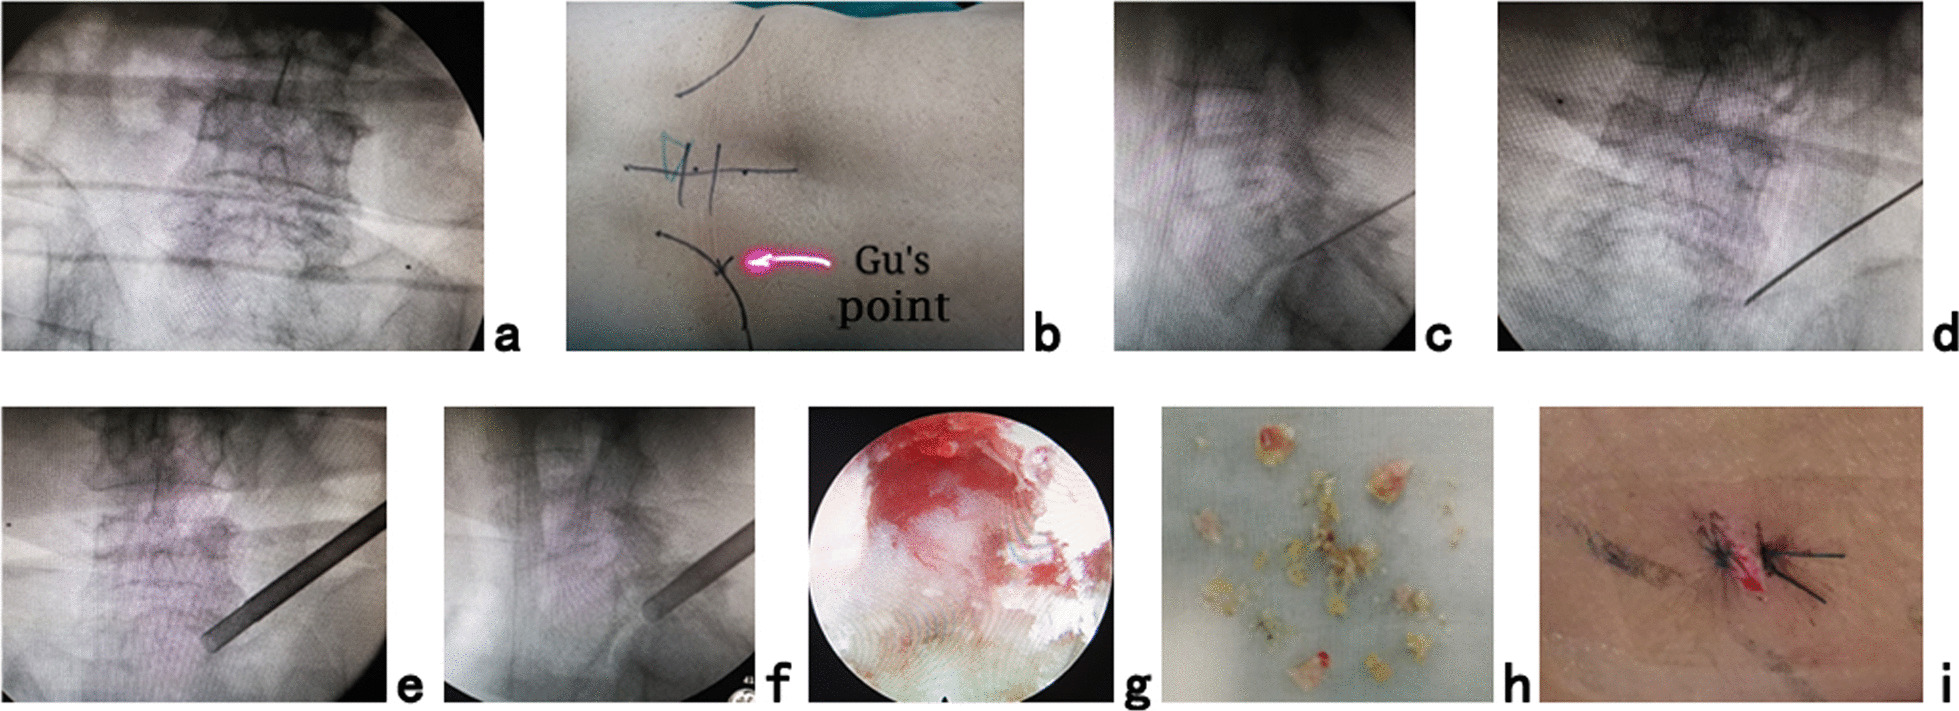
PTES for direct neurologic decompression in the surgical treatment of L5 spondylolisthesis. A transverse line bisecting the disk (L5/S1) was drawn along the metal rod, which was placed transversely across the center of the target disk on a the posteroanterior C-arm view in the prone position. b Photography showed the surface marking of the anatomic disk center identified by the intersection of the transverse line (L5/S1) and longitudinal midline, which was the aiming reference point of puncture, and the entrance point of puncture (Gu’s point) located at the corner of the flat back turning to the lateral side. During puncture, once resistance disappeared, the C-arm view was taken to ensure that the needle reached the target. The tip of the puncture needle was in the intracanal area close to the posterior wall of the disk on c lateral X-ray and near the lateral border of the pedicle on d posteroanterior X-ray. During press-down enlargement of foramen, when resistance disappears, the tip of the reamer should exceed the medial border of the pedicle on the e posteroanterior C-arm view and reach close to the posterior wall target disk on the f lateral C-arm view. Under g the endoscopic view, the compressed nerve root was freed after h the hypertrophic ligamentum flavum and herniated disk were removed. The right leg had a clear sense of relaxation. i The stab incision for PTES was approximately 8 mm (Case 3)
